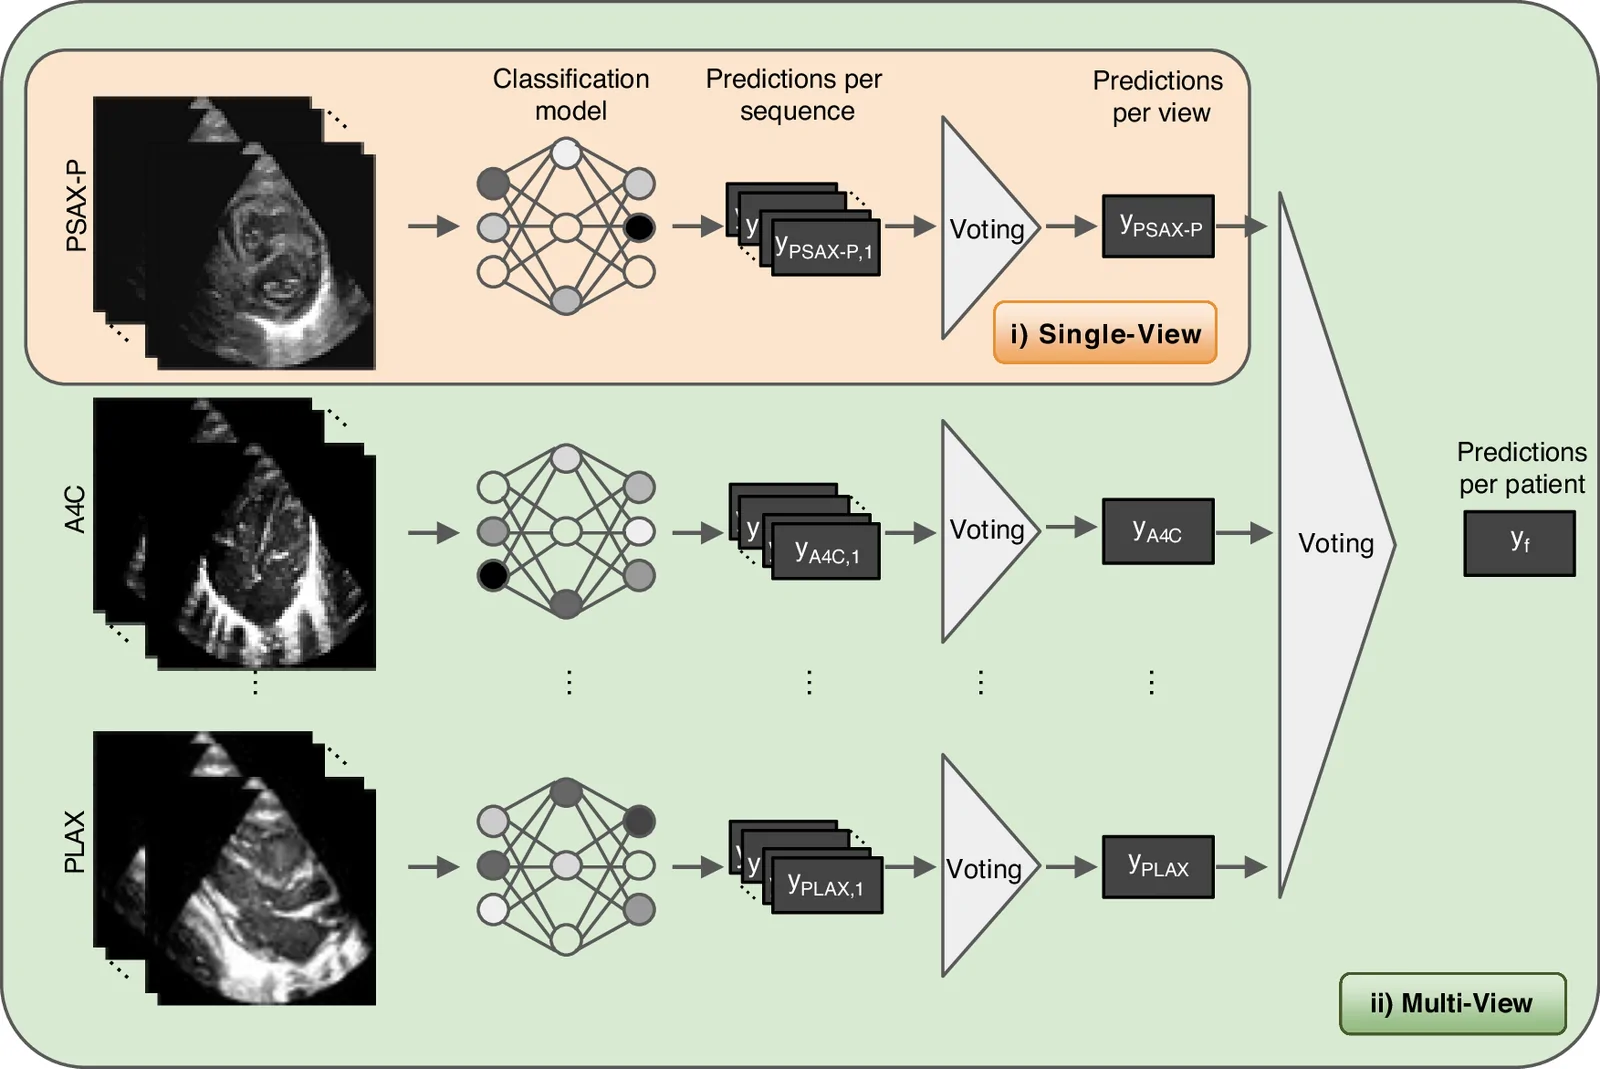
Overview of the automated PH assessment based on spatio-temporal patterns of the echocardiographic videos using majority voting. Panel (i) displays a single view and (ii) a multi-view approach trained on e.g., MV-3 using the three views (PSAX-P, parasternal short axis view; A4C, apical four chamber view; PLAX, parasternal long axis view).
